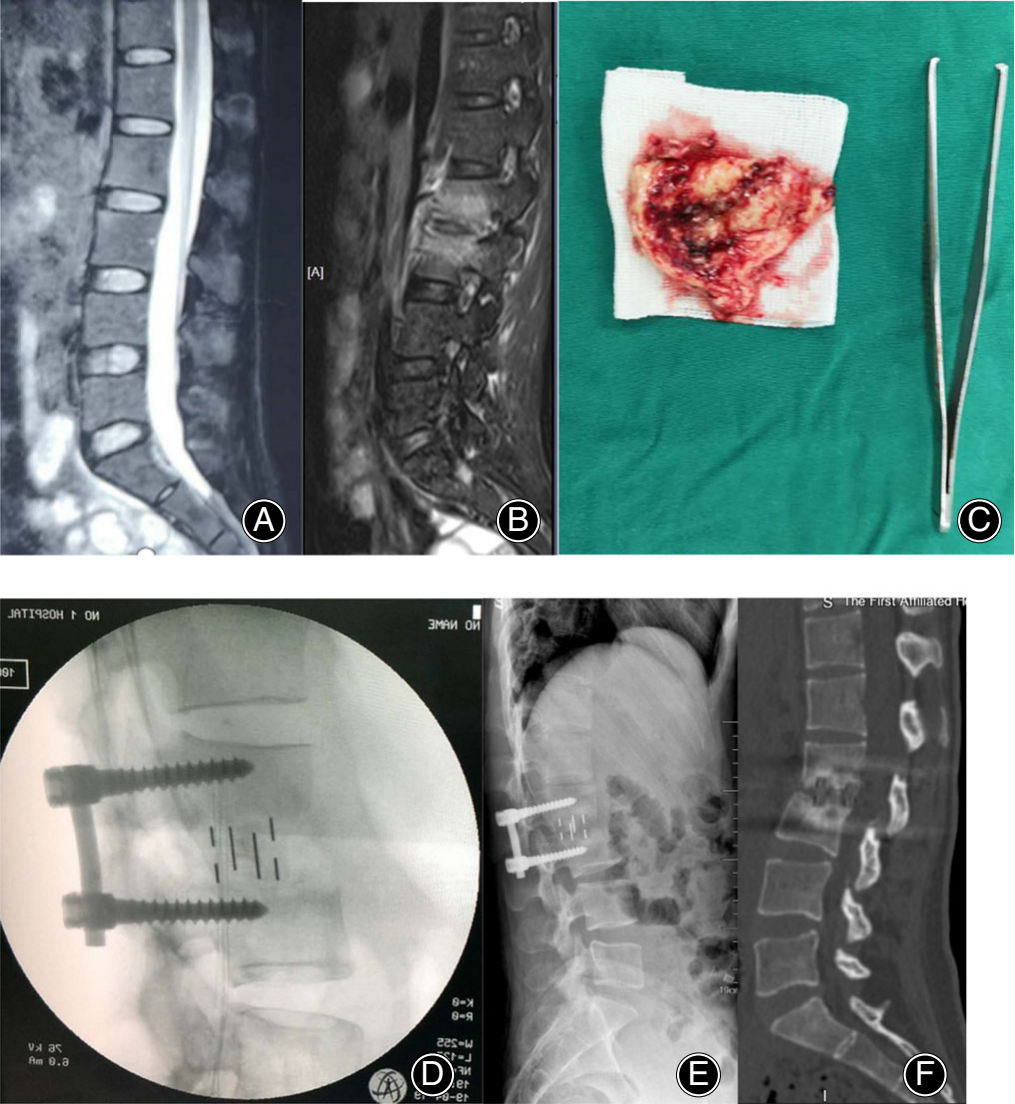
The preoperative sagittal T2‐weighted MRI (A, B) of a 31‐year‐old female patient showed the disruption of intervertebral space 1 week and 2 months after the onset of low back pain, respectively. (C) The disrupted intervertebral disc. The lateral (D–F) radiographs and CT showed oblique lumbar interbody fusion at L2–3 levels intraoperatively, 3 and 6 month postoperatively. Bony fusion was achieved.
